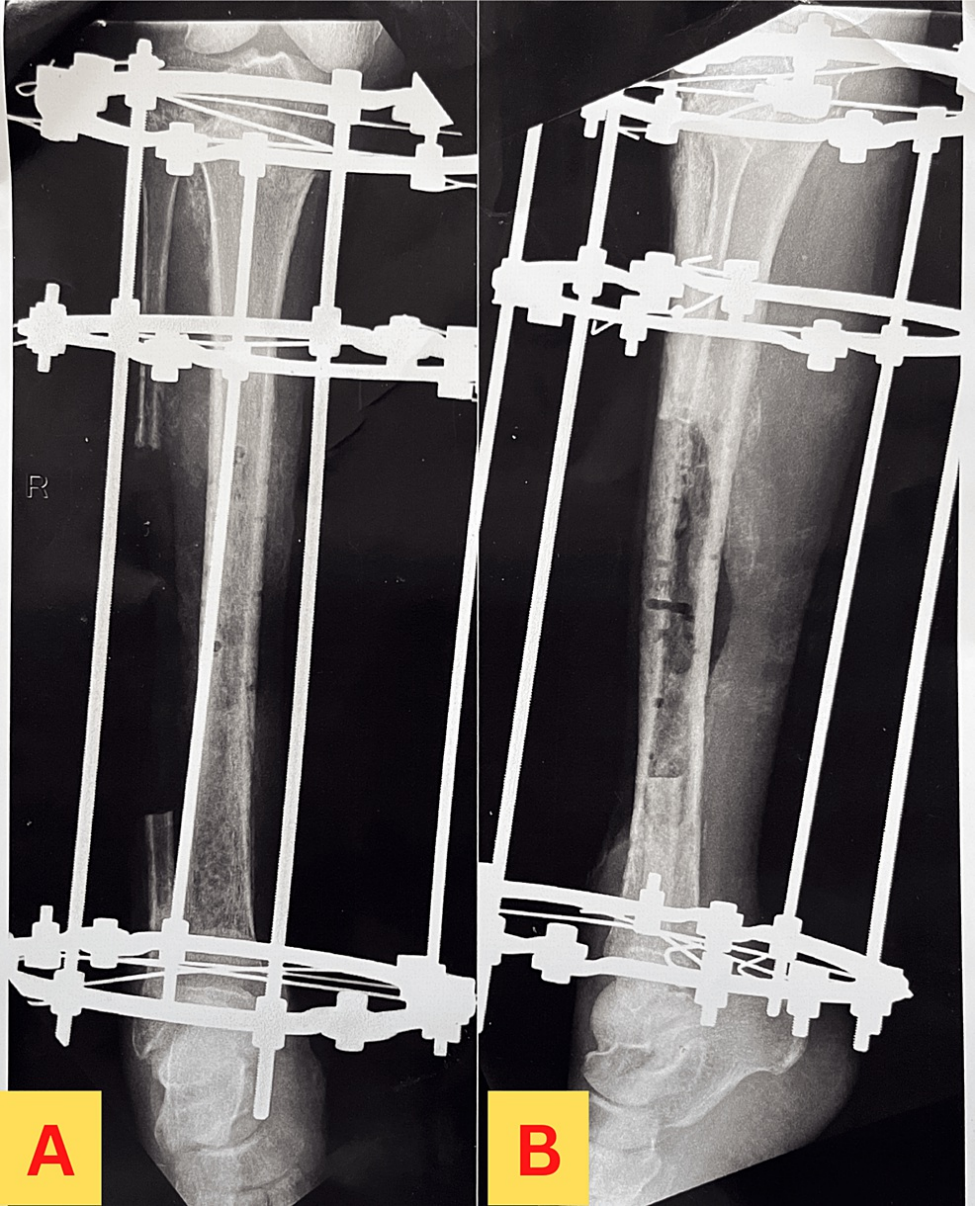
X-ray showing the anteroposterior view and lateral view of the right leg after the application of the Ilizarov fixator. Image A represents the X-ray of the anteroposterior view of the right leg after the application of the Ilizarov fixator. Image B represents the X-ray of the lateral view of the right leg after the application of the Ilizarov fixator.
